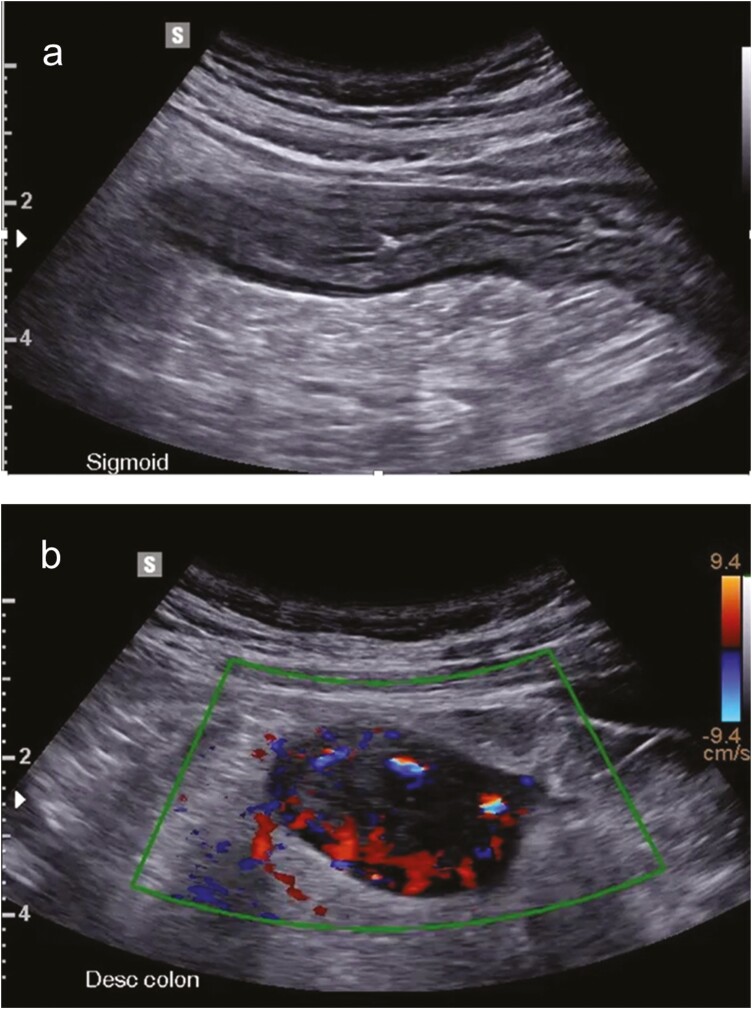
Colonic wall thickening in a patient with active Crohn’s disease (CD). A patient with known colonic CD was assessed at the inflammatory bowel disease (IBD) flare clinic for diarrhea and elevated C-reactive protein in the context of discontinuation of methotrexate due to fear of increased risk of COVID infection. (A) Longitudinal section of the sigmoid bowel with preserved echostratification, thickened wall up to 7 mm, and surrounding echogenic mesenteric fat. (B) Transverse image of the descending colon which is thickened, is hypoechoic, has echostratification loss and with abundant blood flow as seen on color Doppler signal. Images were acquired with a multifrequency convex transducer (3–10 MHz) with a Samsung RS80A machine (Samsung Medison Co. Ltd, Seoul, Korea).
